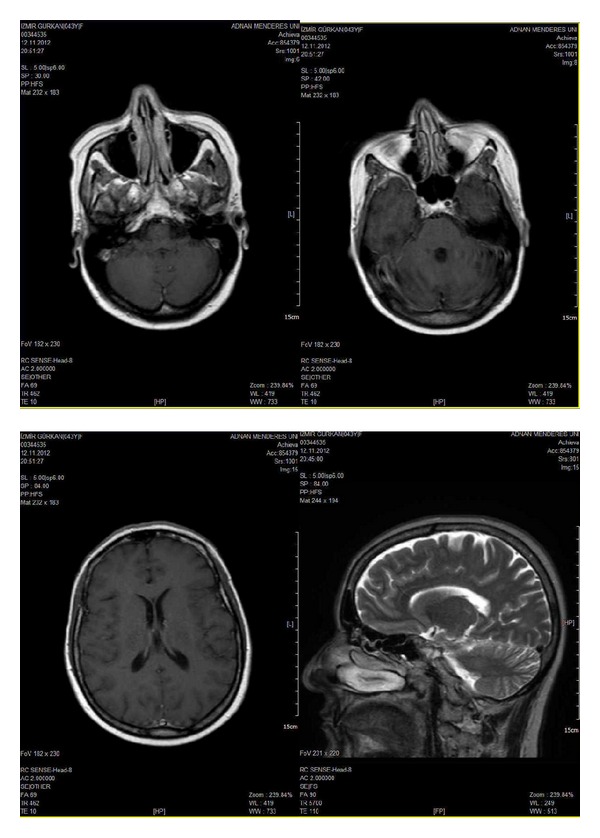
Magnetic resonance imaging scans demonstrating near complete resolution of brain metastases after lapatinib plus capecitabine treatment.
